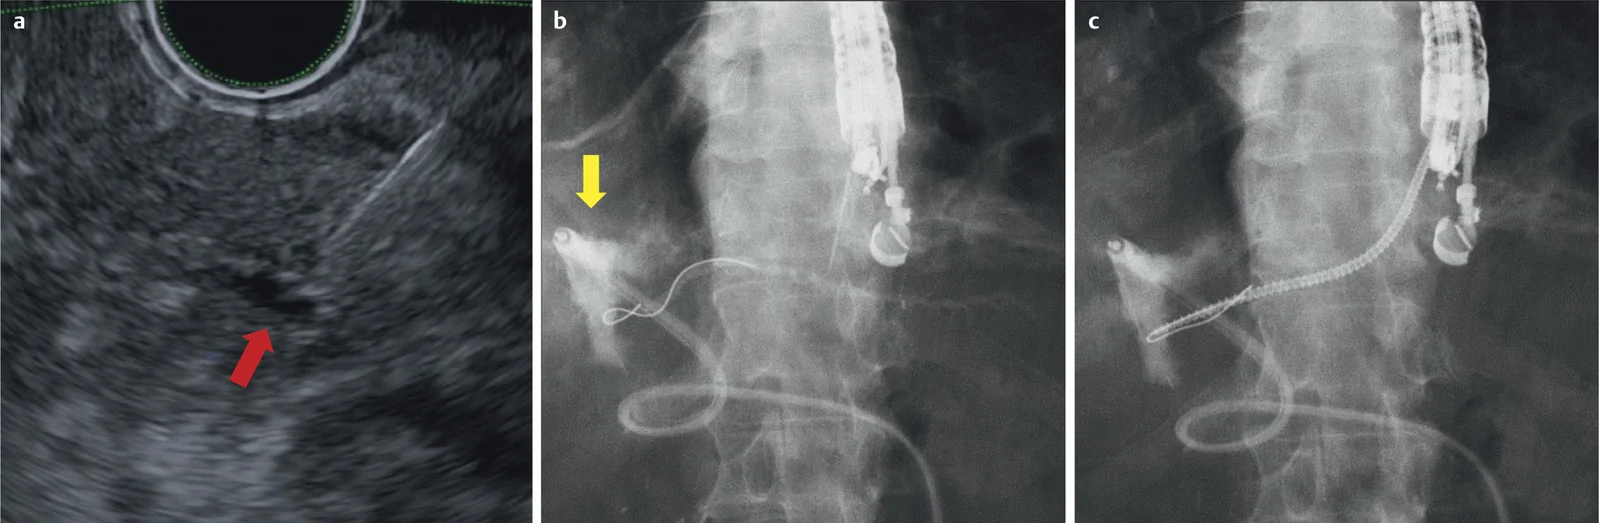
Initial endoscopic ultrasound (EUS)–guided pancreatic duct
